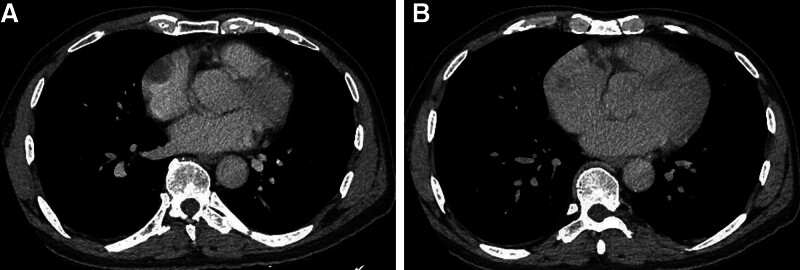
Enhanced CT scan of the chest. (A) After the injection of contrast agent, the right atrium showed a low-density filling defect with an unclear boundary and a lower CT value than the surrounding atrial cavity. (B) The lesion was markedly heterogeneous enhanced on delayed scan, and the maximum cross section was about 3.9 × 2.4 cm. CT = computer tomography.
